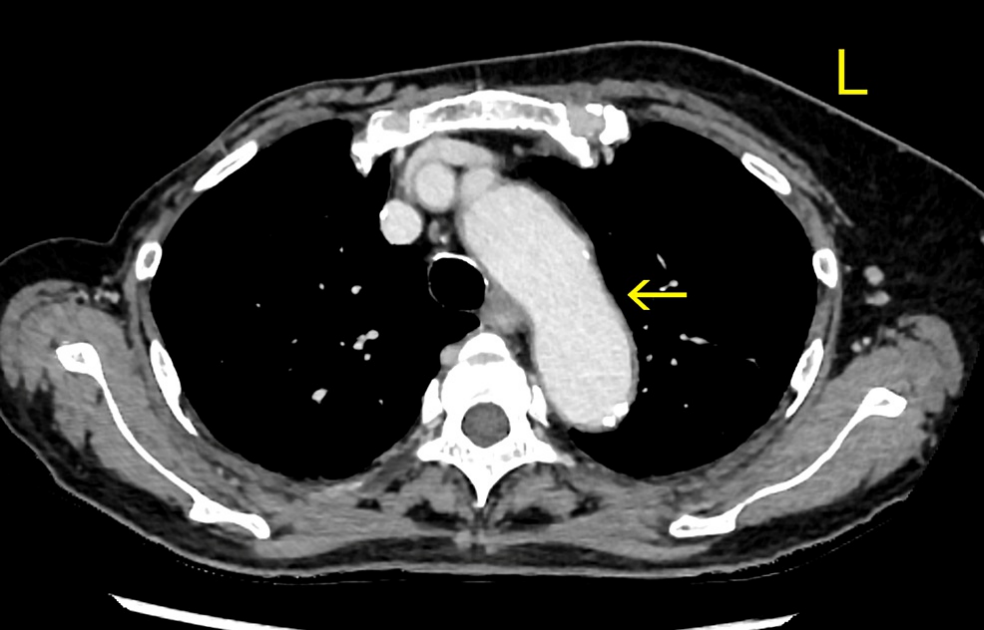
Computed tomography of the chest and pelvis in the first admission. The yellow arrow indicates the aorta; there was no evidence of acute pulmonary embolism and aortic dissection on CT. CT: computed tomography.
